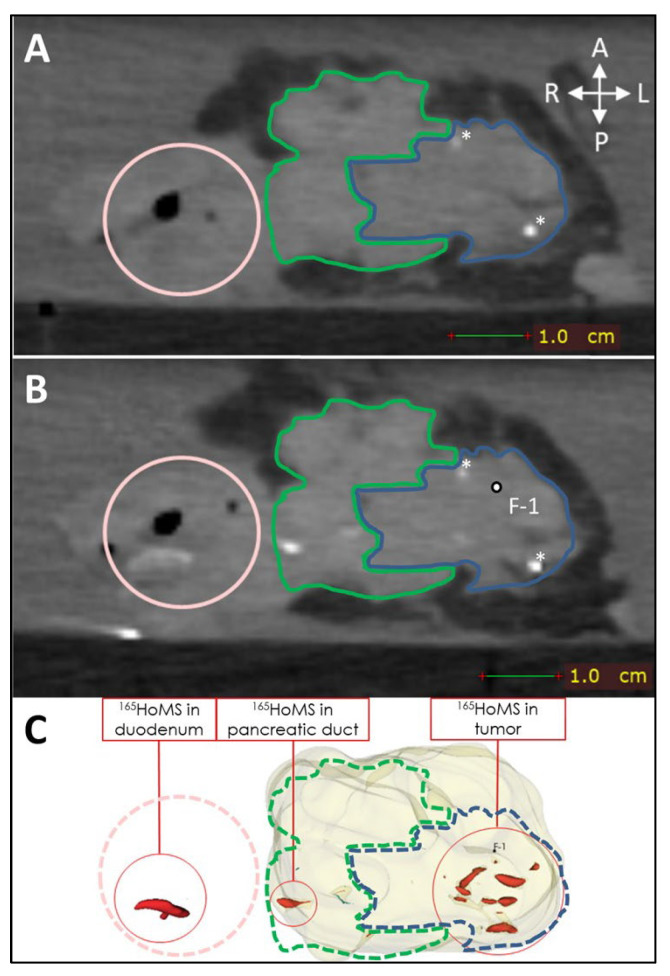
CT (0.5 mm isotropic) of intratumoral ^165^HoMS injection in ex vivo pancreatic cancer sample 9 (vertically aligned). (A) CT before ^165^HoMS injection showing the patient’s anterior (left), posterior (right) (white arrows), duodenum (pink circle), tumor border approximation (blue contour), and healthy pancreas border approximation (green contour). (B) CT scan directly following the injection of 13.4 mg of ^165^HoMSs in a 0.7 mL suspension with the needle removed. Leakage of the ^165^HoMSs occurred from the injection location (F-1, out of plane) through the pancreatic duct toward the duodenum. (C) Three-dimensional segmentation of the pancreatic head (beige), including the healthy pancreas and tumor, and the ^165^HoMSs (red).
